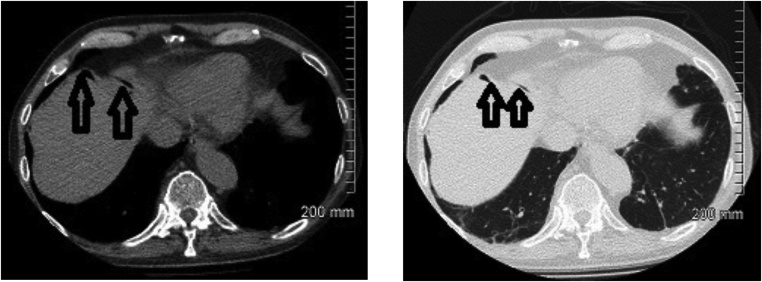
CT thorax showing a right sub-diaphragmatic free air (vertical arrows).
